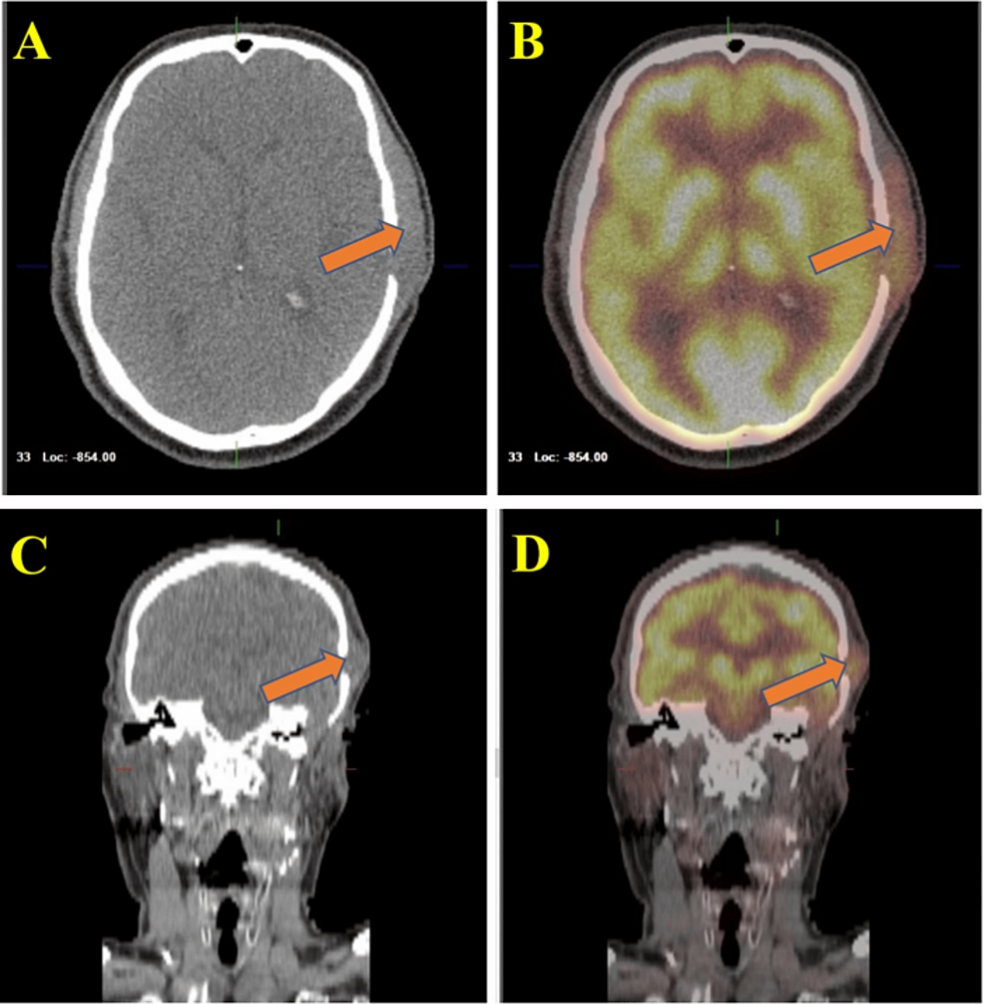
Pre-treatment positron emission tomography-computed tomography (PET-CT) scan of the head and neck Images A and B display coronal views, whereas images C and D show axial views of the PET-CT scan. This scan reveals a region of increased fluorodeoxyglucose (FDG) uptake along the left subcutaneous scalp, which is thickened and measures 6.3 cm in the anteroposterior dimension. The maximum standardized uptake value (SUV) noted is 8.7. There is no clear evidence of FDG avid adenopathy.
